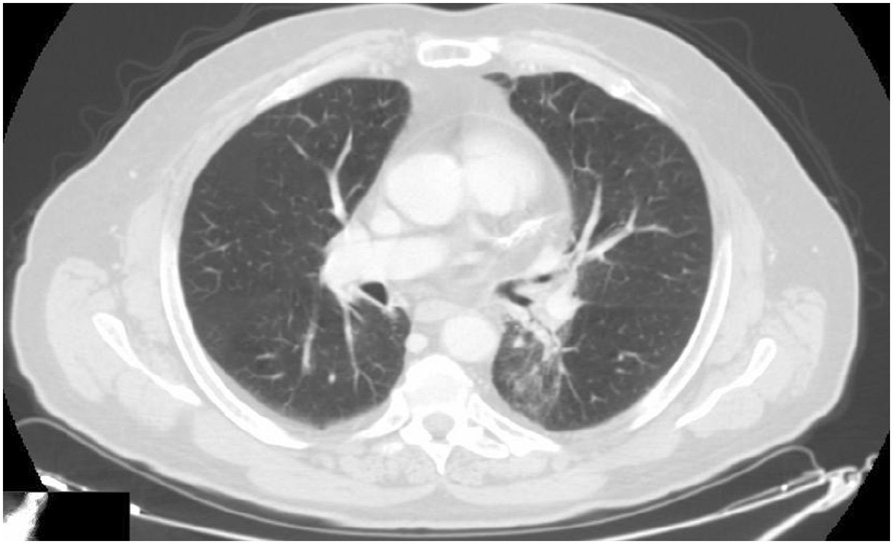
A computed tomography scan performed 8 months after starting treatment with erlotinib.
